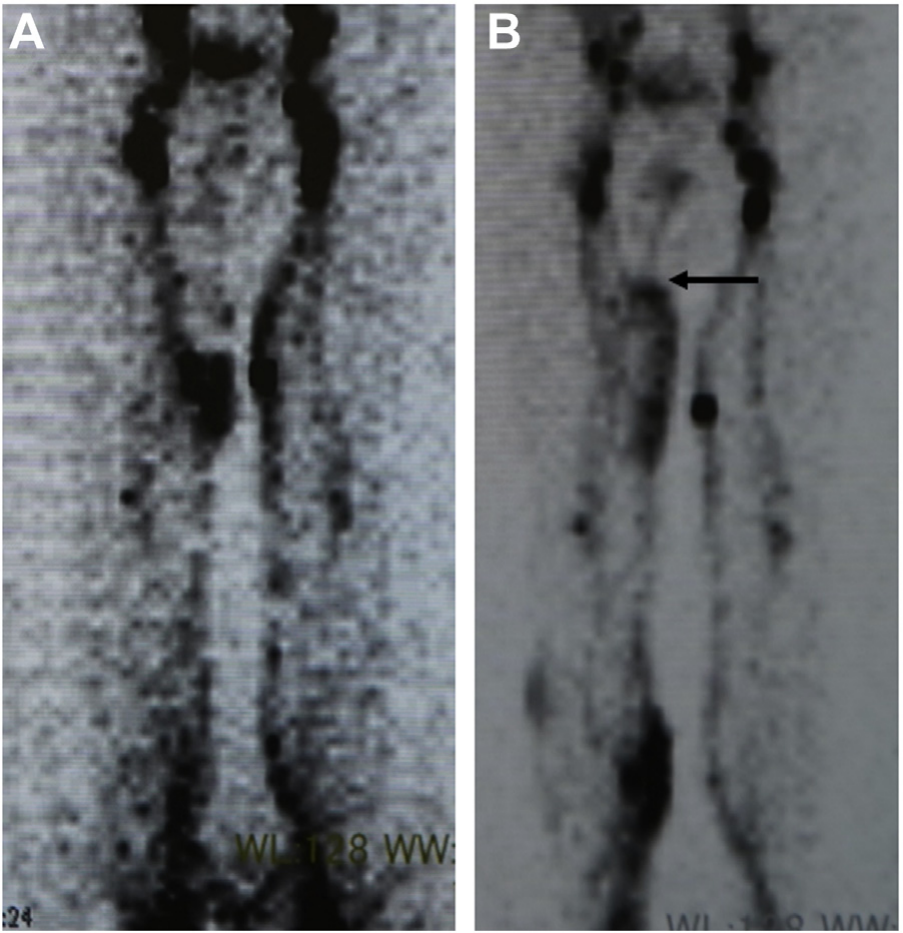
Comparison of lymphoscintigraphy before and after surgery. A, Lymphoscintigraphy before surgery. B, After surgery, lymphoscintigraphy demonstrated upstream lymph flow over the site of the lymphocele, with several pathways found over the proximal side of the right medial thigh (*arrow*).
